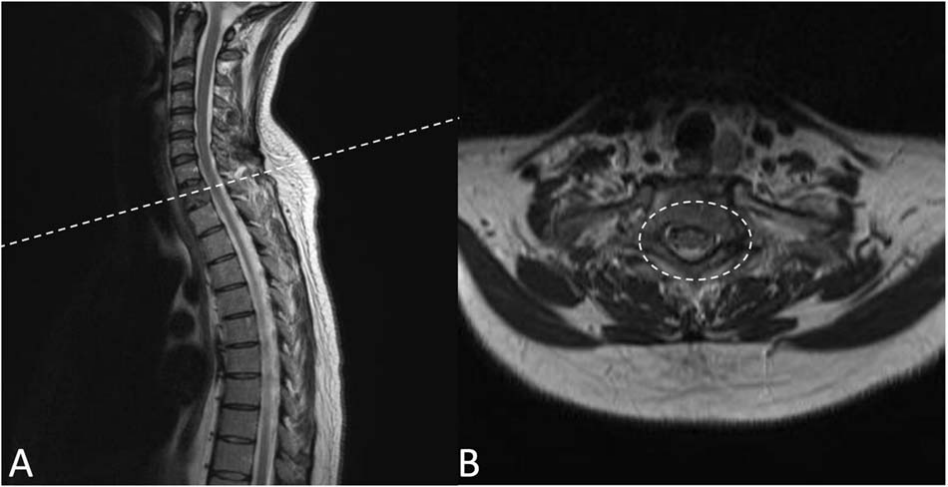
Preoperative MRI Scan December 2019. (A) Sagittal view of oligometastatic lesion at T2 vertebral body. (B) ESCC grade 2 determined as there is spinal cord compression, but with cerebrospinal fluid visible around the cord.
